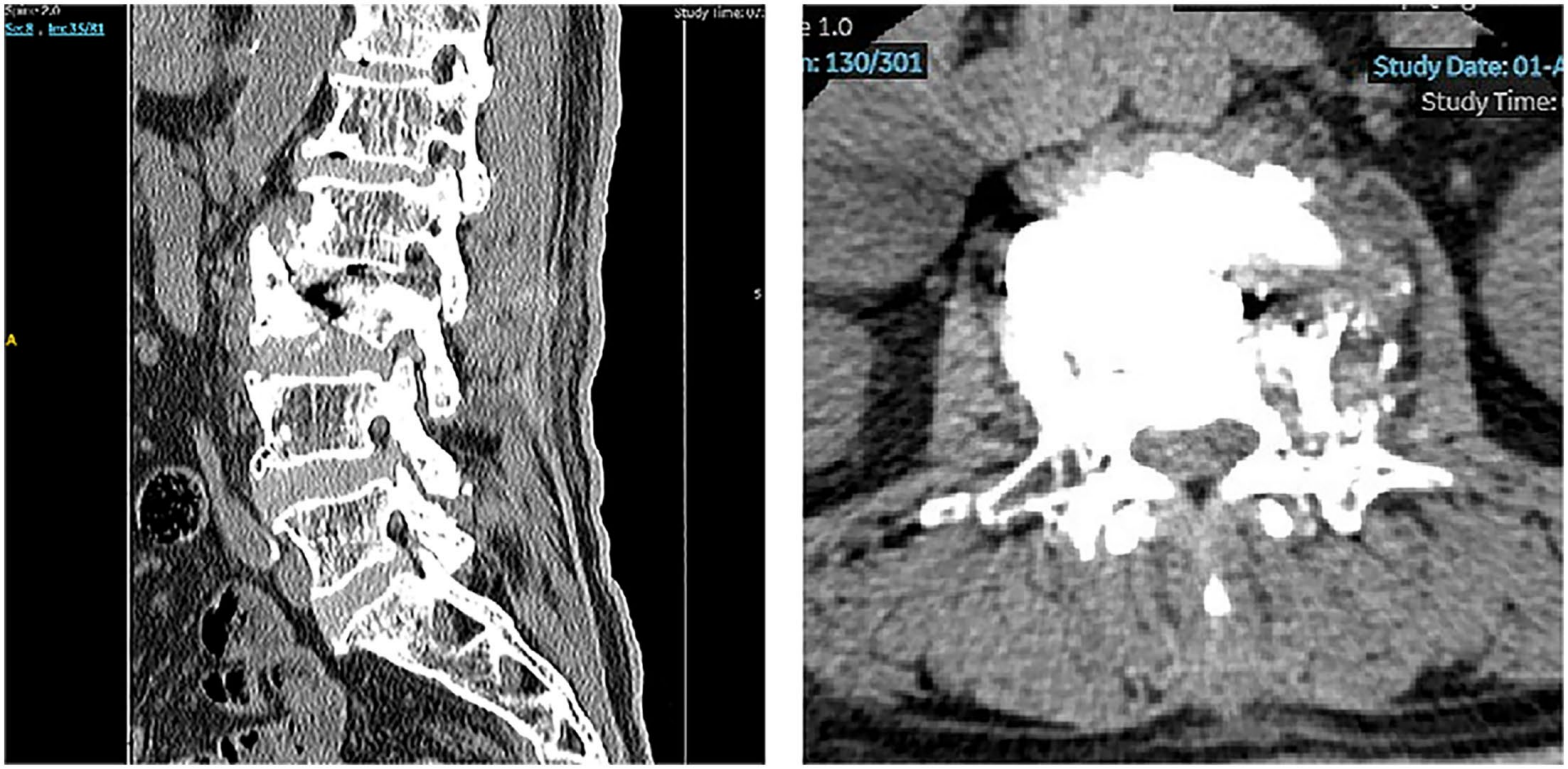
Postoperative lumbar radiographs and CT. Cement is satisfactorily placed within the L3 vertebral body and the L2/3 intervertebral disk. Postoperative imaging demonstrates resolution of the focal kyphotic deformity, restoration of normal spinal alignment, and complete decompression of the L2/3 neural foramen and lateral recess.
